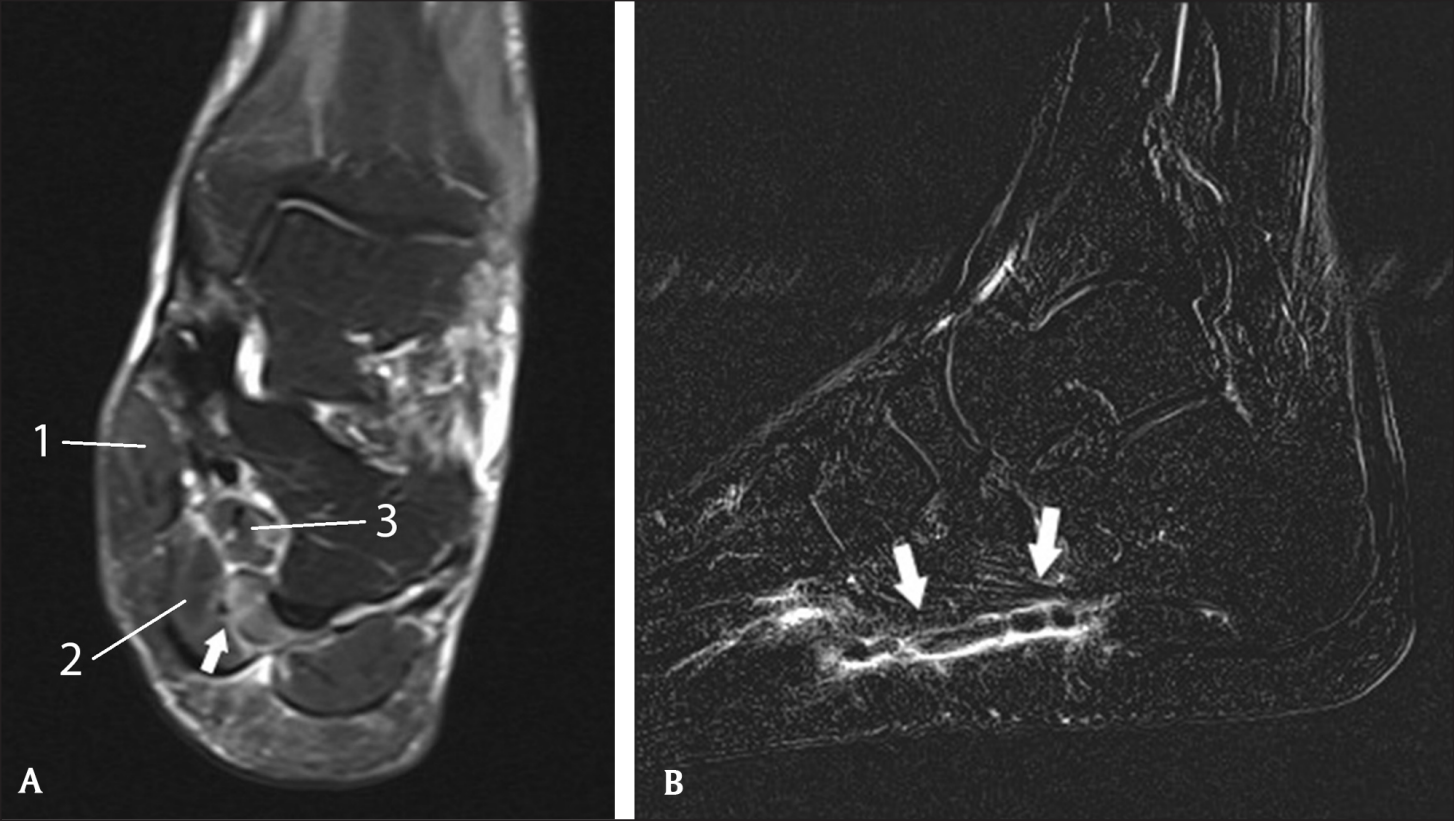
MRI of the left foot. Coronal reformatted T2-WI FS (A) showing subtle intramuscular edema (arrow) adjacent to the medial plantar veins. The thrombosed vein itself is not visible. Medially from the medial plantar artery and veins is the m. abductor hallucis (1); laterally the m. flexor digitorum brevis (2); and deeply beneath is the m. quadratus plantae (3). Sagittal reformatted T1-weighted subtraction image after gadolinium (B). There is a filling defect in the medial plantar veins (arrows) with a thin sleeve of hyperintense contrast around the thrombus.
